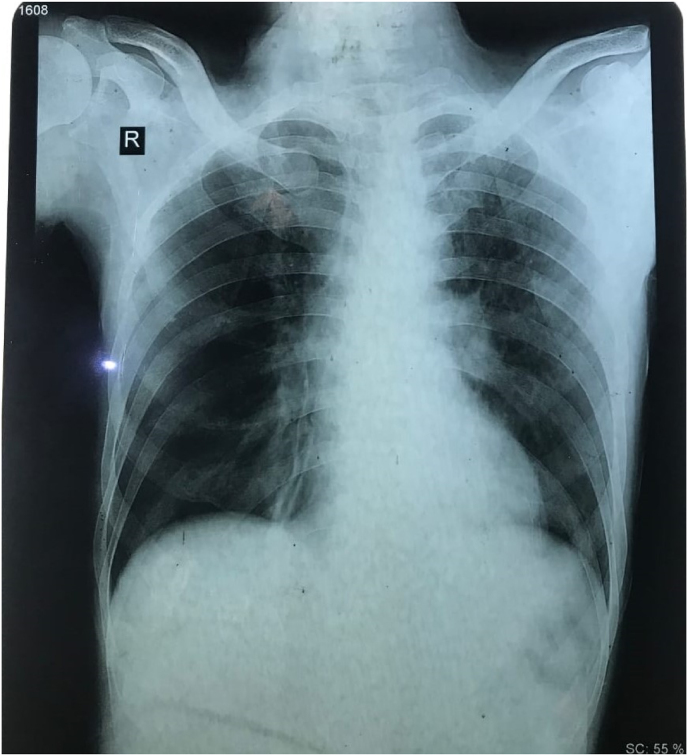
Chest x-ray revealing no significant findings; normal.
